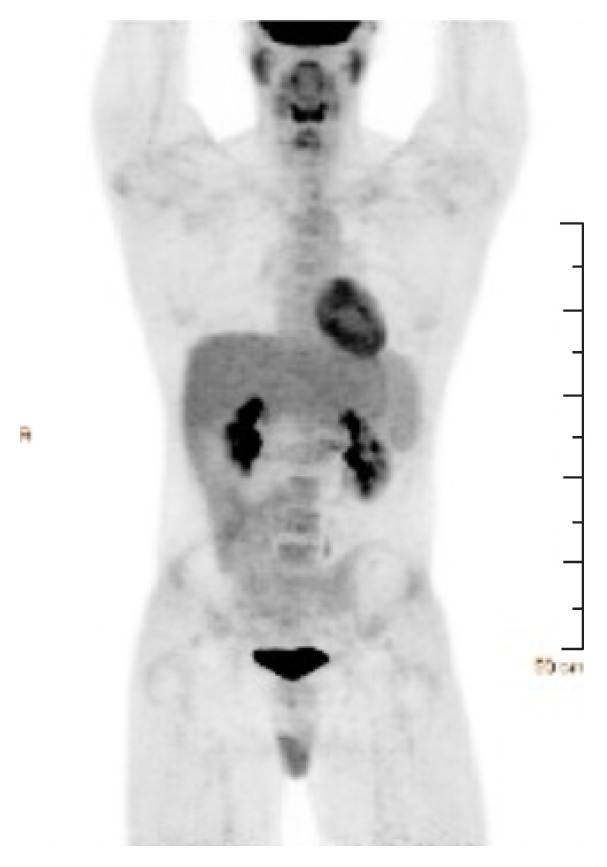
PET scan at Cycle 3/Day 1 showed interval resolution of FDG avid lymphadenopathy and bone lesions.
